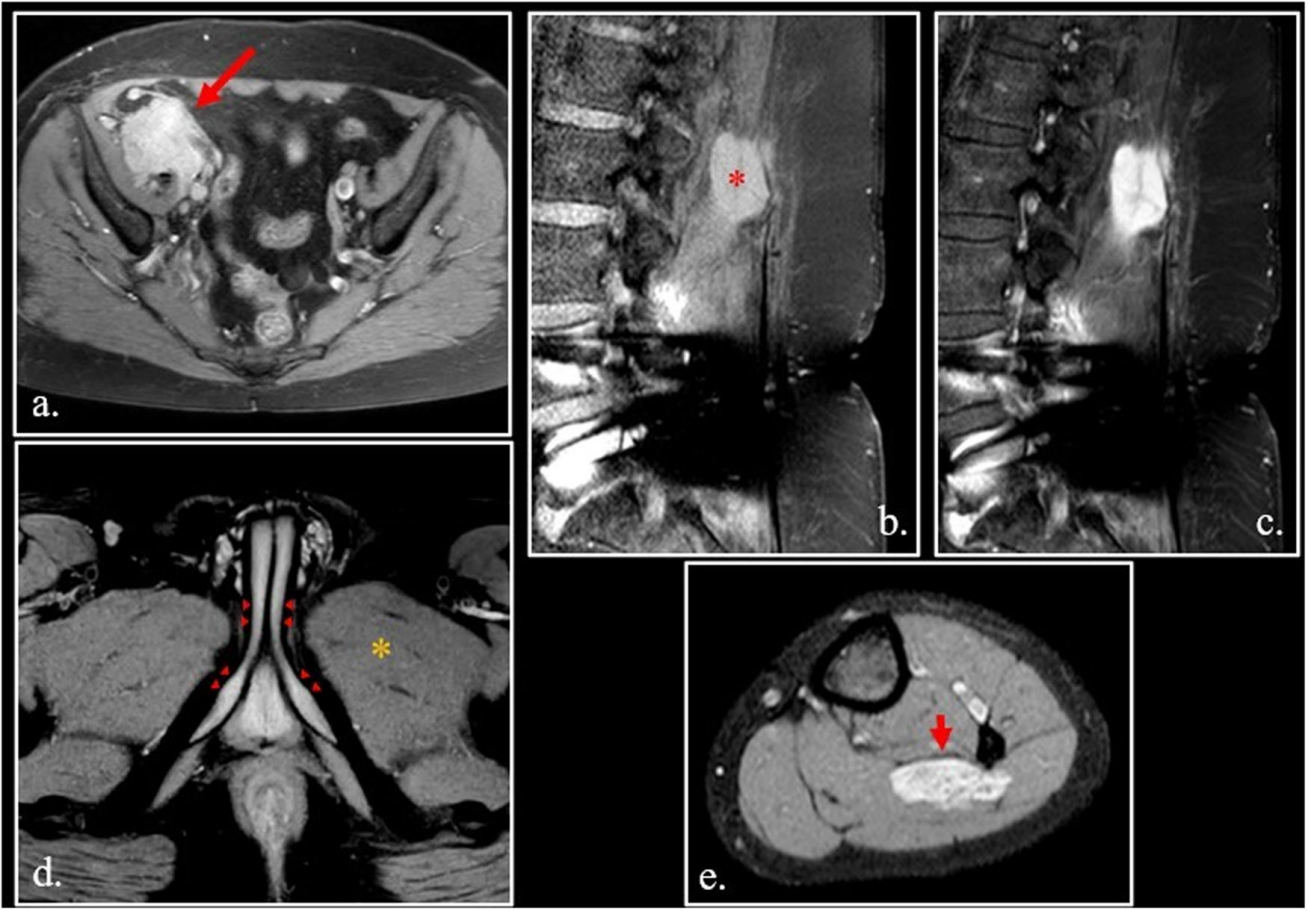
a FSE T1-weighted fat-sat of the lower abdomen shows a hyperintense lymphoma (arrow) in the right iliac fossa. b, c Unenhanced (b) and enhanced (c) sagittal FSE T1-weighted fat-sat images of hypercellular desmoid of paraspinal muscles (asterisk). The lesion is strongly hyperintense on unenhanced T1-weighted scan due to high cellularity. d Unenhanced FSE T1-weighted fat-sat image. The signal intensity of the corpora cavernosa (arrowheads) is quite higher than that of muscles (asterisk). e Unenhanced FSE T1-weighted fat-sat image shows a strongly hyperintense hemangioma (arrow) of the soleus muscle
